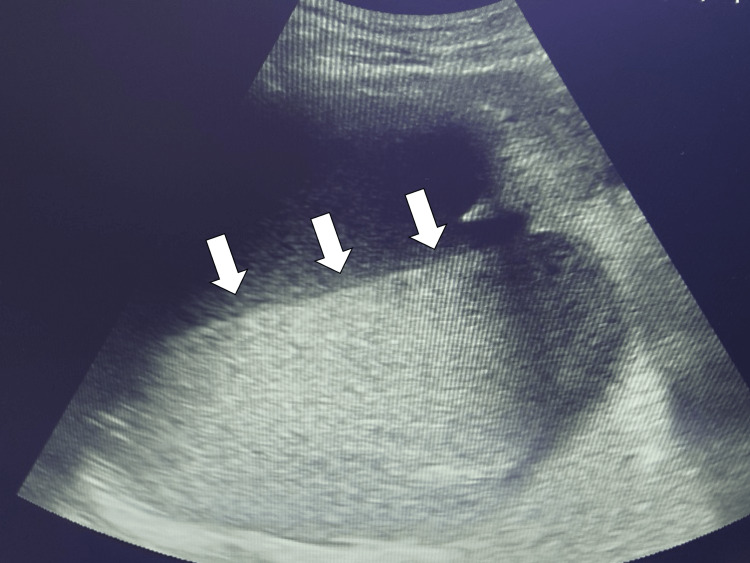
Abdominal ultrasound imaging showing debris in the hepatic cyst
